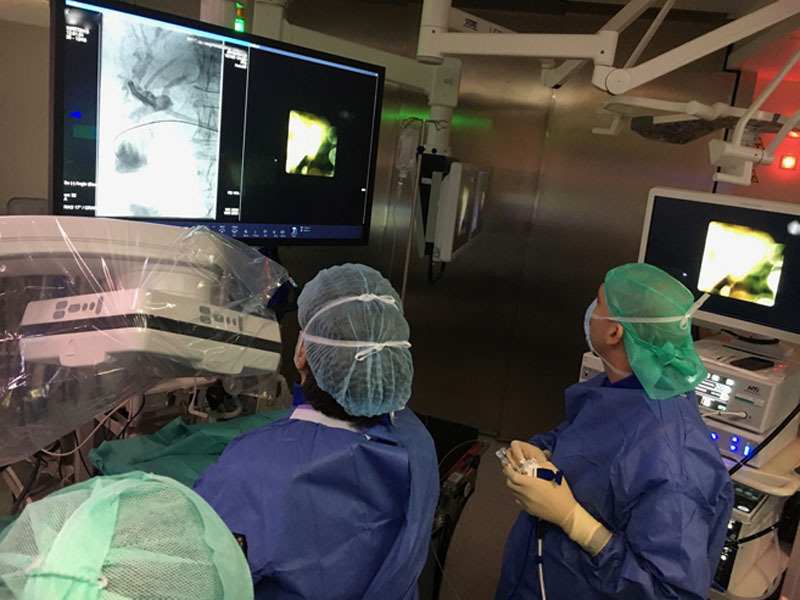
Collaboration between a biliopancreatic endoscopist and an interventional radiologist in the case of a patient with a previous total gastrectomy and large common bile duct stones. A SpyGlass direct visualization system (Boston Scientific, Marlborough, USA) was introduced percutaneously, and electrohydraulic lithotripsy was performed until complete stone clearance. High-definition medical imaging systems (computed tomography, cone beam computed tomography, and magnetic resonance imaging) combined in the same suite allowed the use of real-time and 3-dimensional imaging to show the absence of residual stones (ARTIS Pheno, SOMATOM, and MAGNETOM systems; Siemens Healthineers, Forchheim, Germany).
